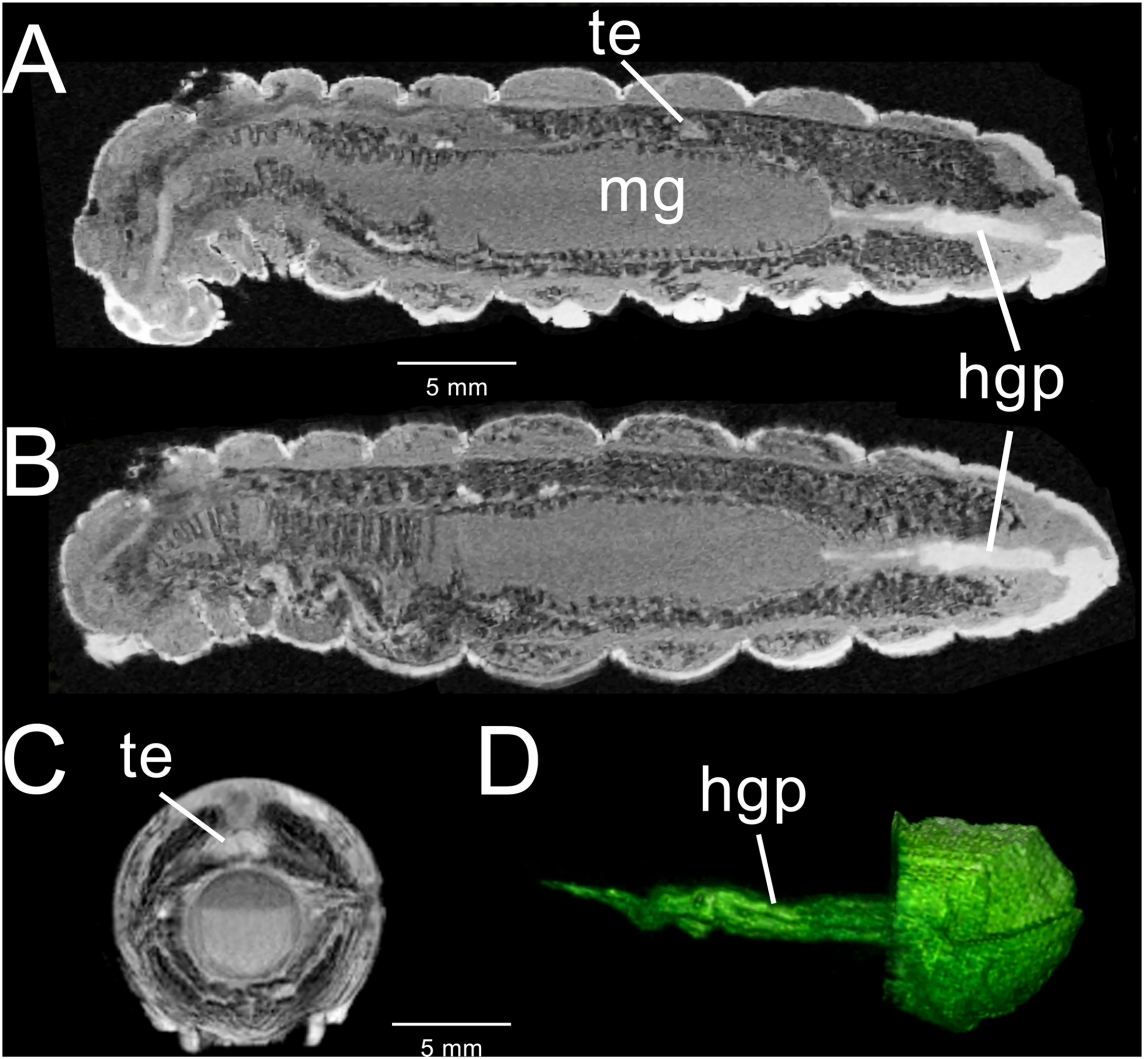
3D-GRE MR imaging data acquired from a male fifth instar *M*. *sexta* 12–16 hours before metamorphosis. (A) Mid-sagittal section displaying the testes (te), hindgut “plug” (hgp) and highly enlarged midgut. (B) Coronal section. (C) 2D-SE image showing a transverse section through the abdominal segment at the level of the testes. Layering in the midgut (mg) is clearly shown. Neither layer appears to contain gas bubbles. (D) Ventral 3D surface-rendered view of the hindgut “plug” and terminal abdominal segments.
